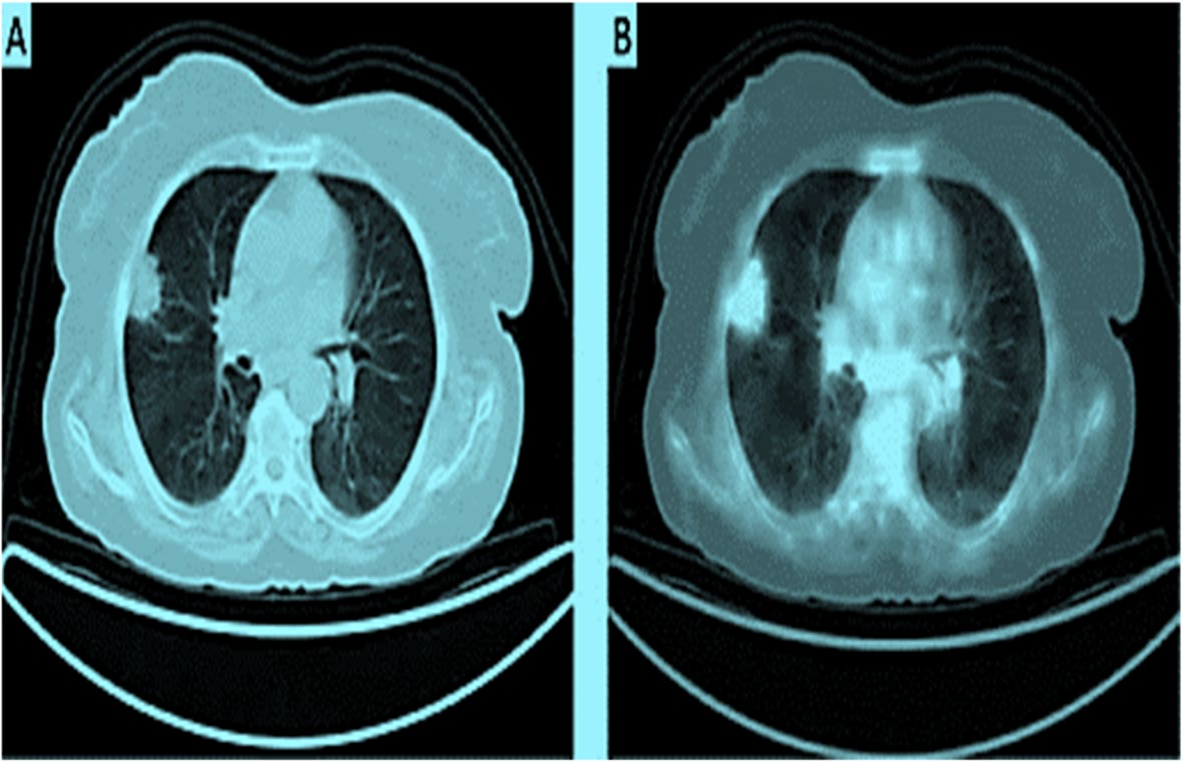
A A CT scan reveals a nodule in the anterior portion of the right lung's upper pole. B On 18F-FDG PET/CT, the lung nodule exhibited enhanced focused uptake, indicating a malignancy [82]
